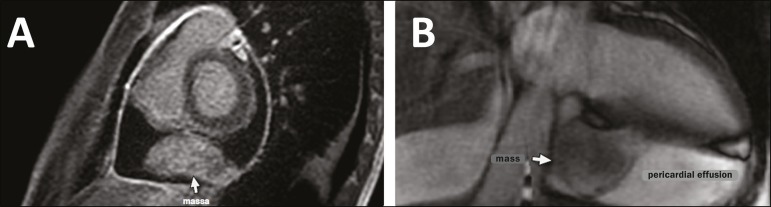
Cardiac MRI with delayed enhancement, in short-axis and long-axis views
